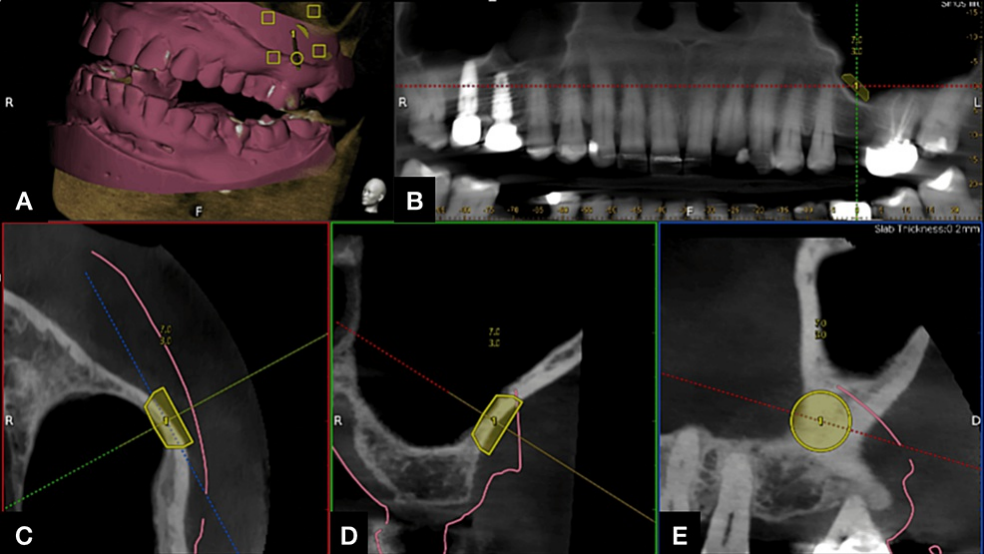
Lateral window trajectory planning under the dynamic navigation software (Navident) (A) Cone beam computed tomography (CBCT) data and stereolithography (STL) files merged into the software. (B) Lateral window opening planning in the orthopantomogram (OPG) view. (C, D, E) Lateral window planning in the occlusal, bucco-lingual, and mesio-distal view
